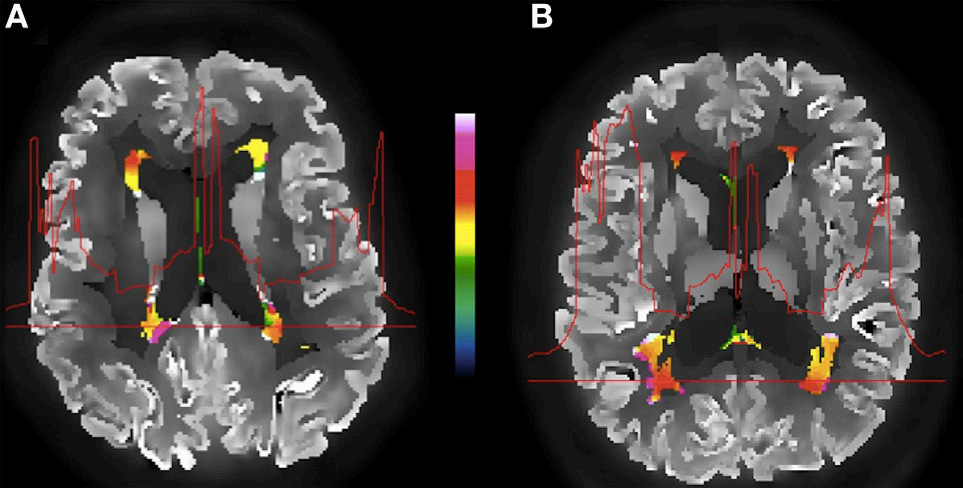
Example of an amyloid-positive (A) vs. amyloid-negative (B) subject and the corrected FDG-PET images, WMH segmentation (color), and the metabolic profile (red line) for the selected WMH section across the brain. The color bar indicates normalized relative uptake.
